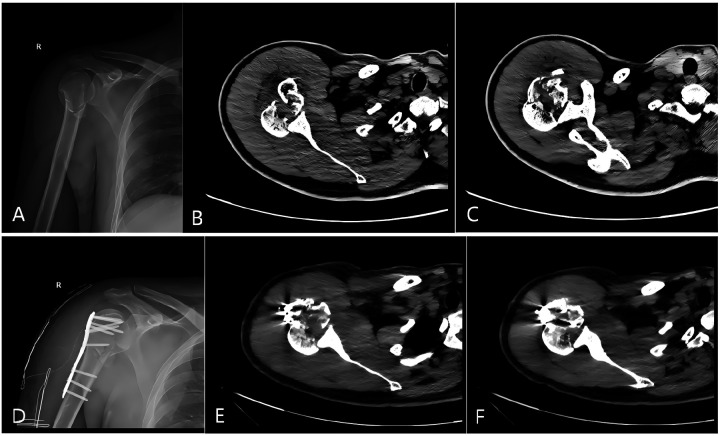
(A) Anteroposterior radiograph at the initial presentation shows a proximal humeral fracture with the “lightbulb sign”. (B,C) CT scans obtained at the initial presentation demonstrate a four-part proximal humeral fracture with loss of humeral head–glenoid congruency, a locked posterior dislocation of the humeral head, and a RHSL. (D) Anteroposterior radiograph after the initial surgery still shows the “lightbulb sign”. (E,F) Postoperative CT scans show poor humeral head–glenoid alignment, and the locked posterior dislocation was not corrected.
